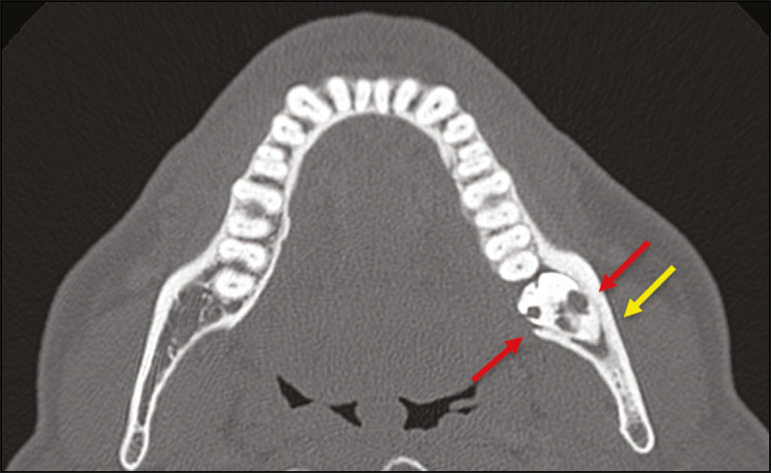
Axial CT of the paranasal sinuses showing lower left third molar (tooth 38) partially unerupted, impacted at the distal root of the second molar, showing cariogenic lesions (red arrows) together with signs of periodontitis (yellow arrow).
